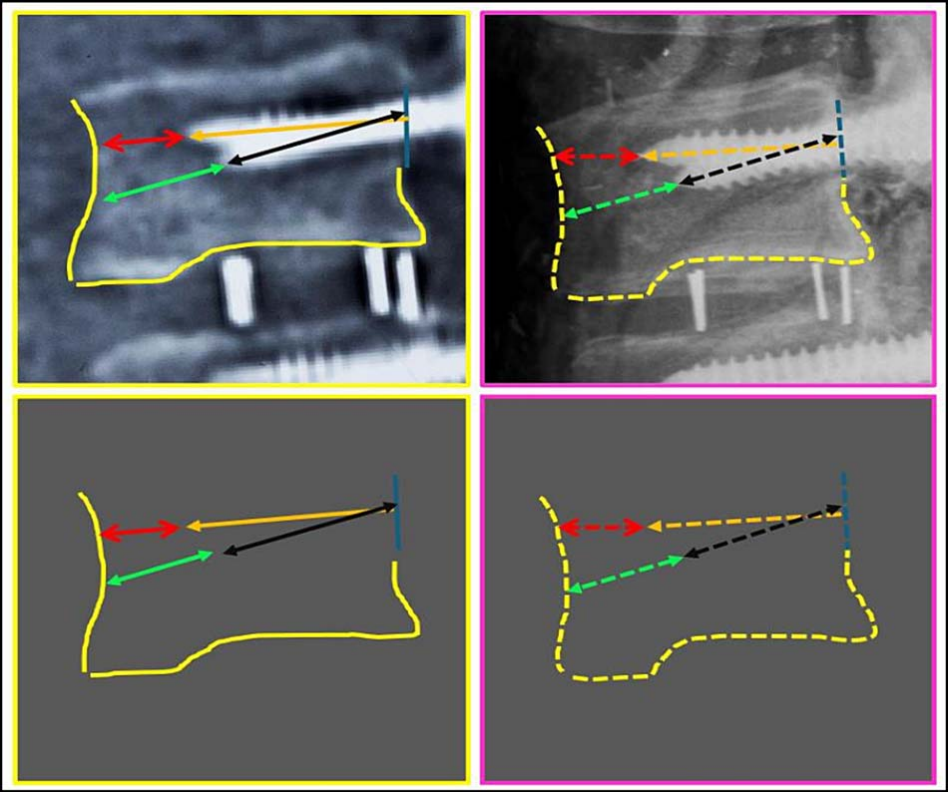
L3 vertebral body. Upper panels, comparison between the antemortem CT images (left) and postmortem lateral X-ray (right). Lower panels, respective concordant templates.
